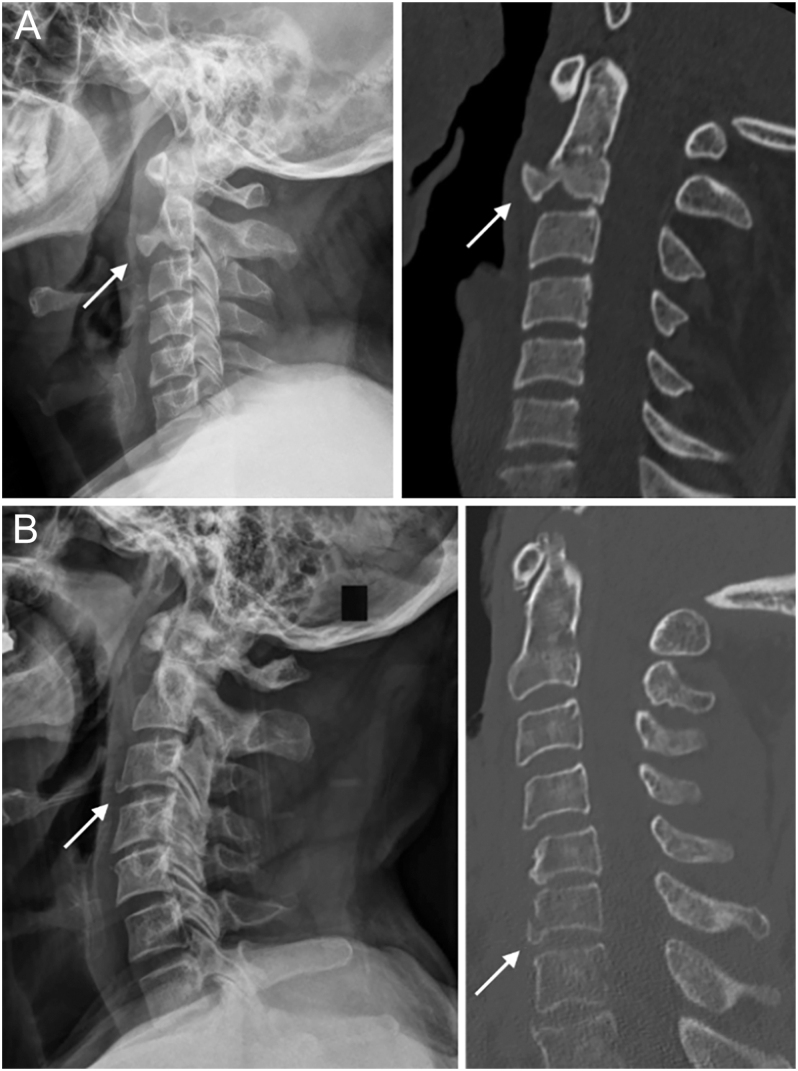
(A and B) Extension teardrop fracture of C2. Left: X-ray, right: CT. (C and D) Subaxial extension teardrop fracture. Left: X-ray, right: CT.
